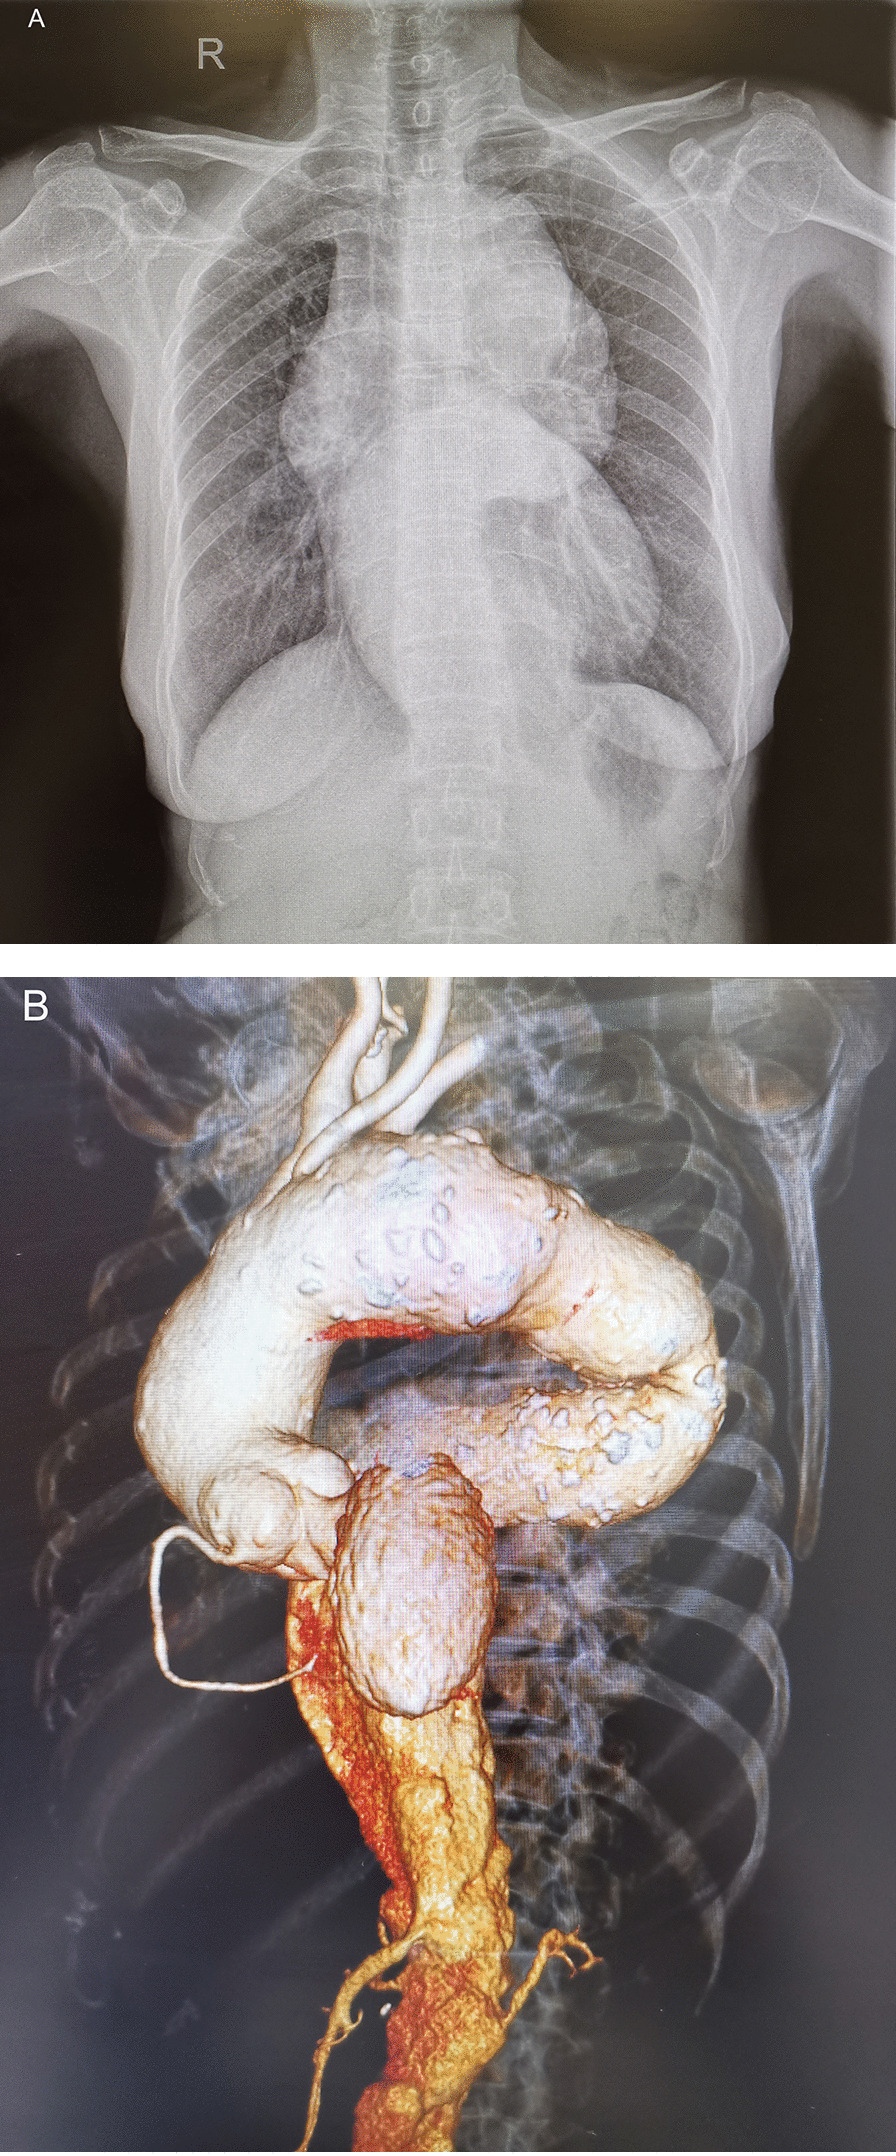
Chest radiograph showed a huge mass in the upper mediastinum (A); CTA showed a total aortic aneurysm, and the lesion involved the aortic root, ascending aorta, aortic arch, and the entire thoraco-abdominal aorta. The aneurysm is tortuous and deformed, mural thrombus can be seen in the aortic arch and abdominal aorta, multiple penetrating ulcers can be seen also in the thoracic and abdominal aorta, and the widest part of the aneurysm is 72 mm in diameter (B)
